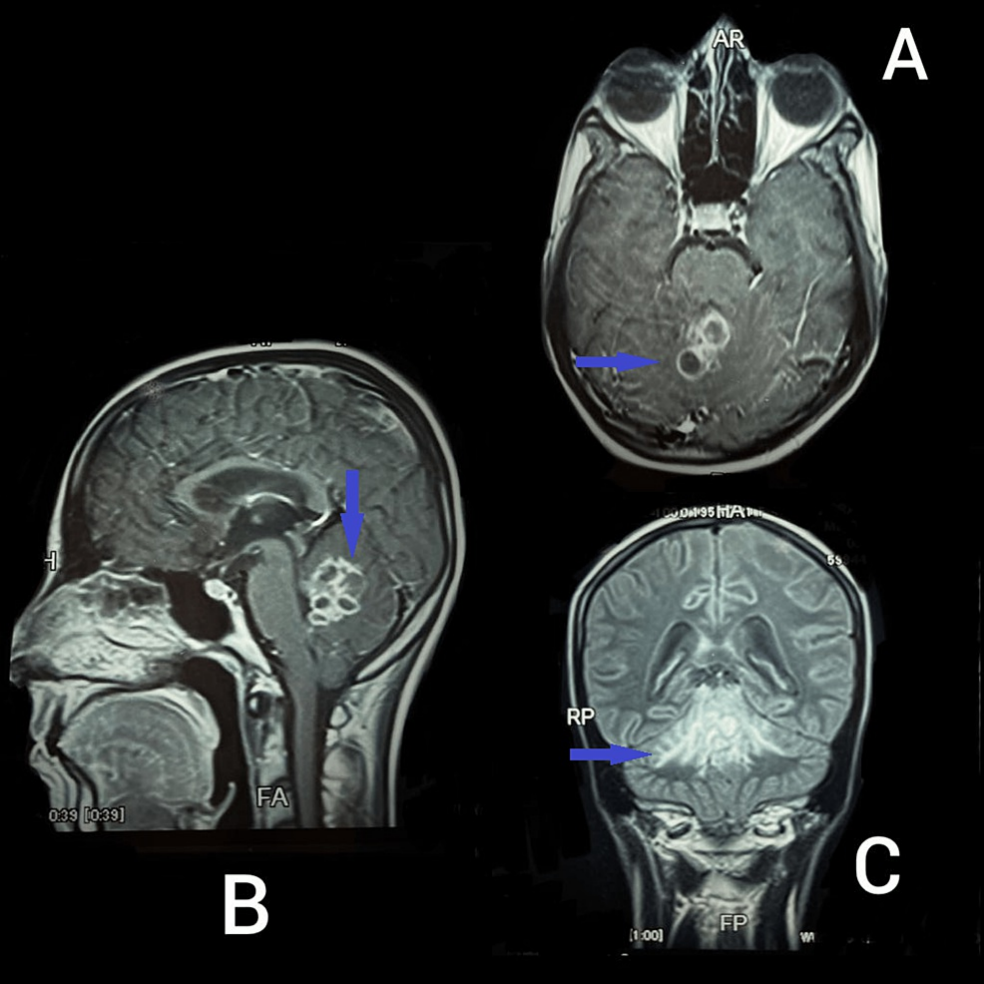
MRI of the brain A: Axial view showing peripheral ring-enhancing confluent lesions (arrow) in cerebellar vermis on post-contrast imaging. B: Sagittal view showing conglomerate mass (arrow) seen in cerebellum on post-contrast imaging. C: Lesions appearing hyperintense on fluid-attenuated inversion recovery (FLAIR) imaging with surrounding edema (arrow) in coronal view.
